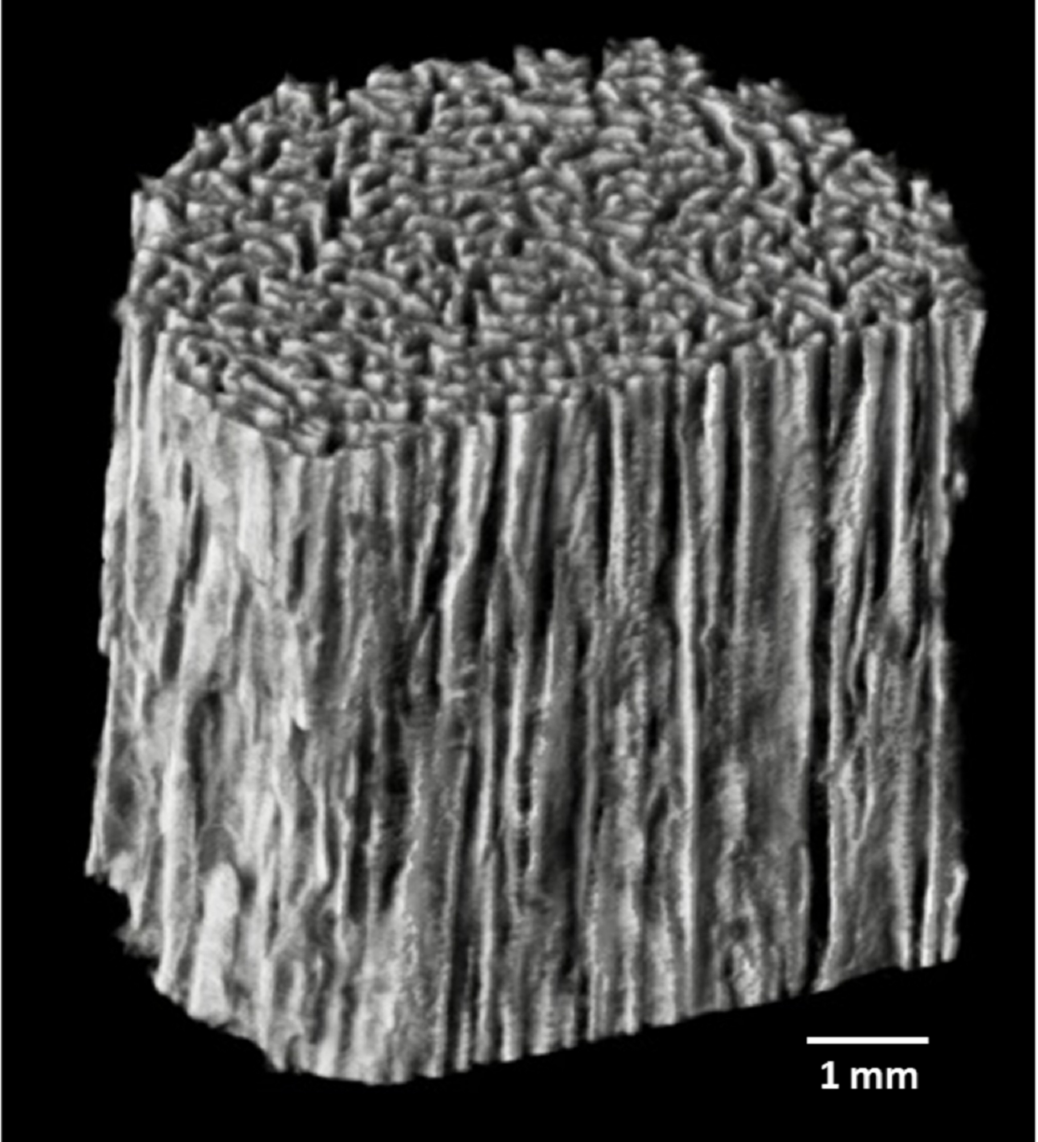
3D-CT image of Affinos Affinos has a unique, characteristic structure in which unidirectional pores 25-300 μm in diameter are aligned in one direction. Affinos: Kuraray Co., Ltd., Tokyo, Japan
